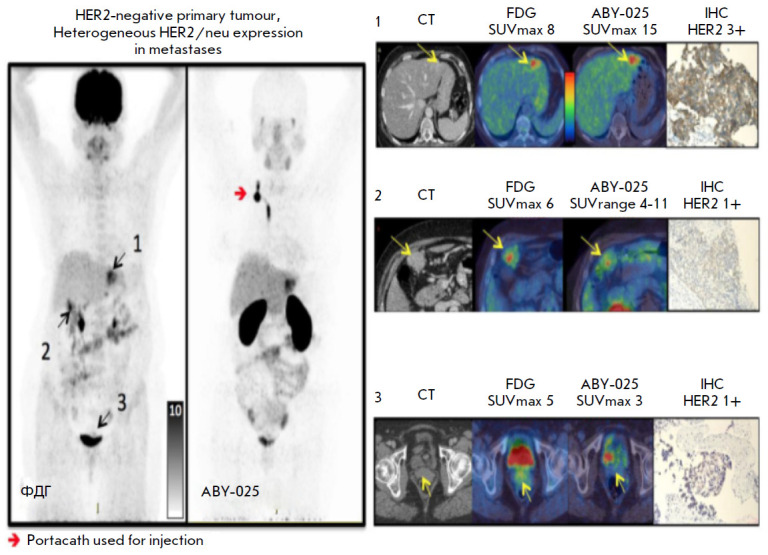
A patient with HER2-negative primary breast cancer. FDG-PET/CT detected
